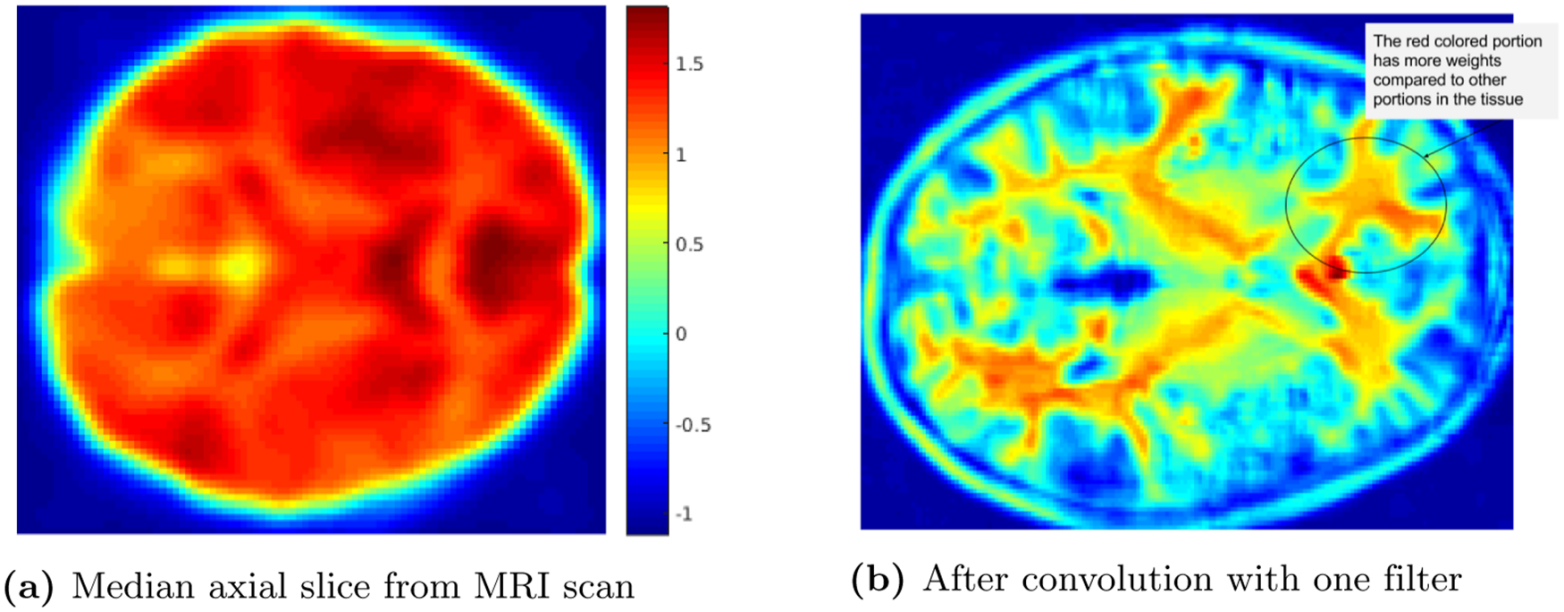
(a) Median axial slice from MRI scan and (b) the result after convolution with a filter.
